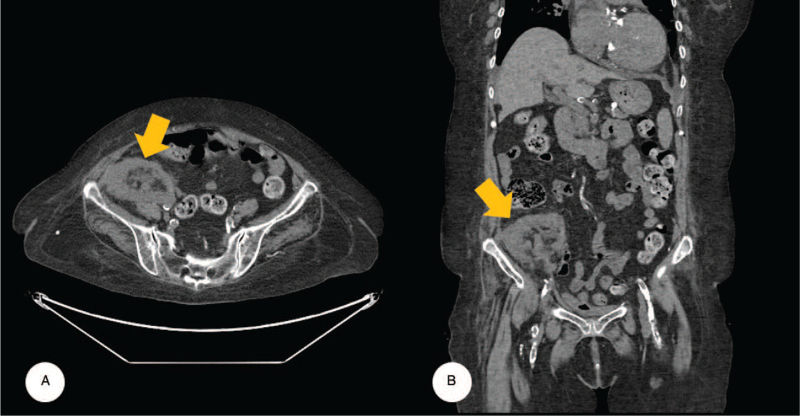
(A) Transverse, and (B) coronal views of the abdomen-pelvis computed tomography demonstrated mild atrophic change in the transplanted kidney at the right iliac fossa (arrows).
